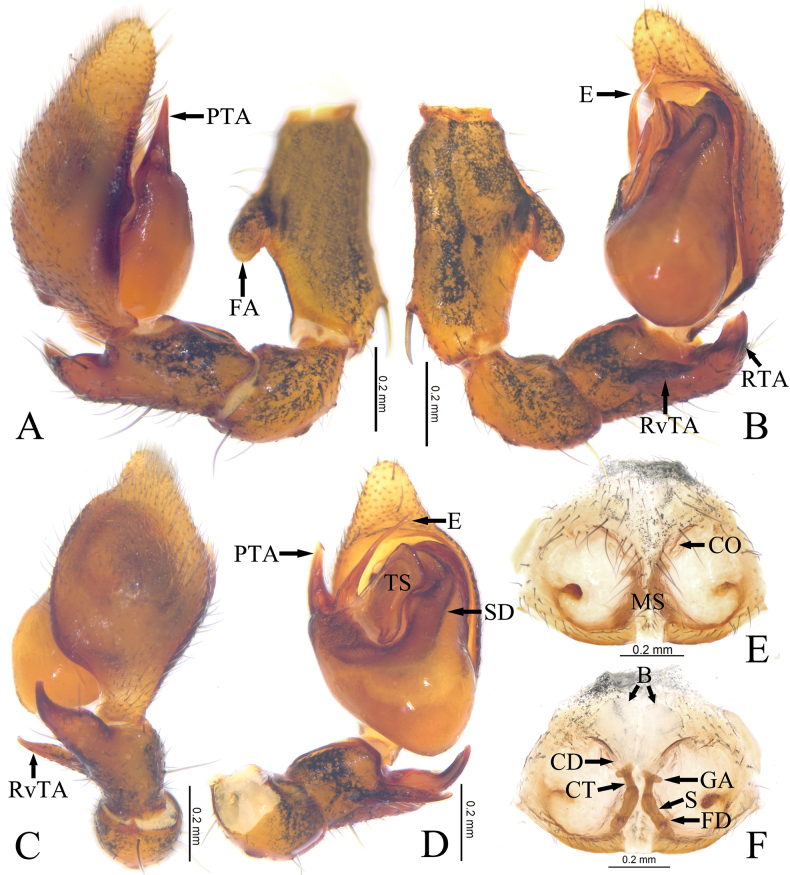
Copulatory organs of *Mutatustujia* sp. nov. A male left palp, prolateral view B same, retrolateral view C same, dorsal view D same, ventral view E epigyne, ventral view F same, dorsal view. Abbreviations: E—embolus; FA—femoral apophysis; PTA—prolateral tegular apophysis; RTA—retrolateral tibial apophysis; RvTA—retroventral tibial apophysis; SD—sperm duct; TS—tegular sheet; B—bursa; CD—copulatory duct; CO—copulatory opening; CT—connecting tube; FD—fertilization duct; GA—glandular appendage; MS—median septum; S—spermathecae.
